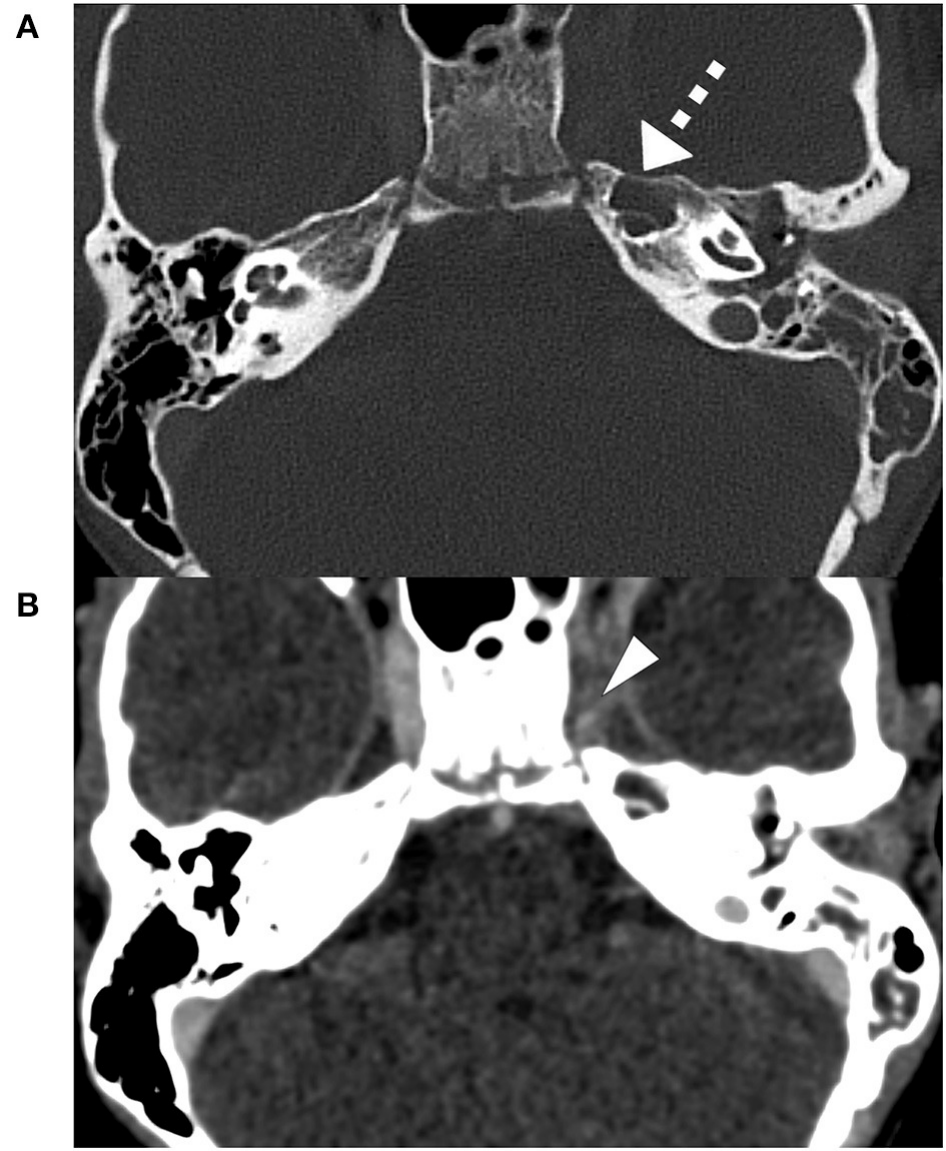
Initial contrast-enhanced CT-Scan bone window, slice thickness 0.75 mm: partially obstructed mastoid air cells with a fully opacified, pneumatized petrous apex (triangle) on the left side (A). Initial contrast-enhanced CT-Scan soft tissue window, slice thickness 0.75 mm: Obstruction of the left cavernous sinus (dashed arrow), suspicious of cavernous sinus thrombosis (B).
